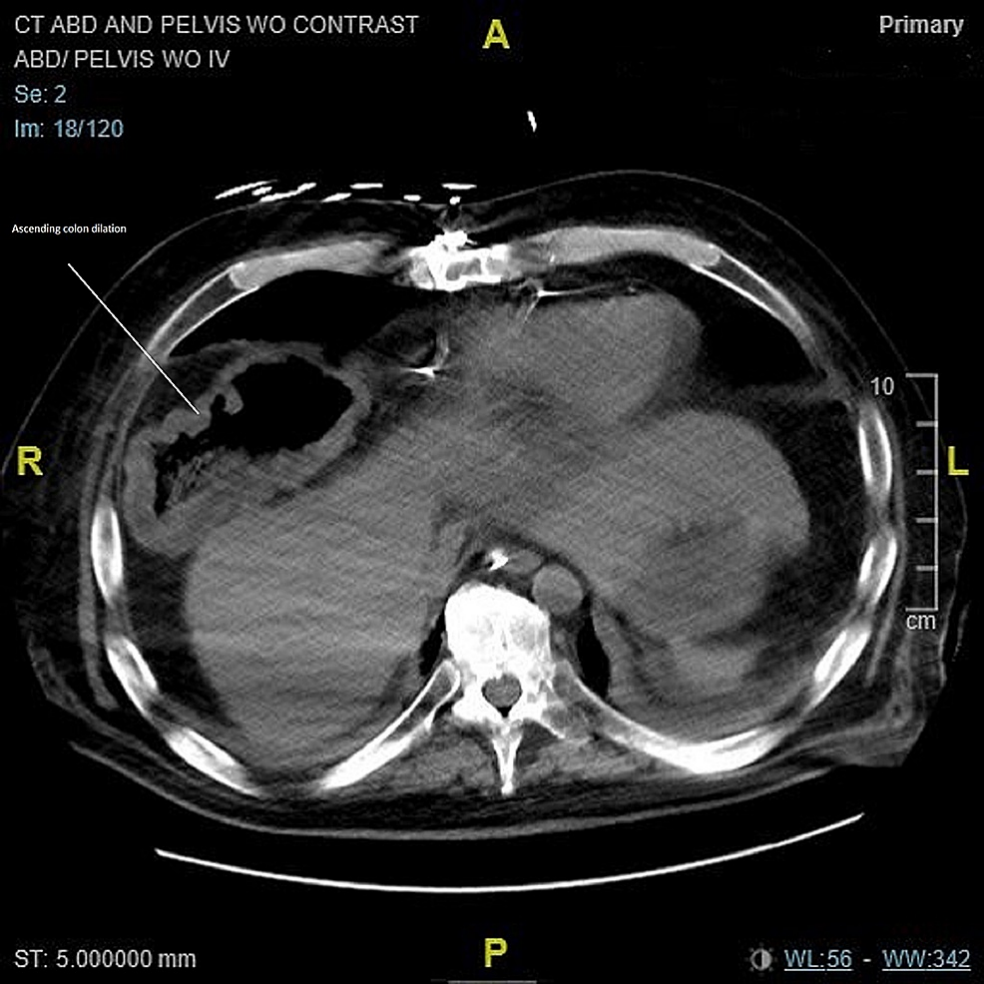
CT of the abdomen/pelvis on worsening of infection. Axial view: Circumferential thickening of the colon wall most marked within the right colon. Increased colonic wall thickening concerning for progression of infection. Before the use of fidaxomicin. CT: computed tomography
